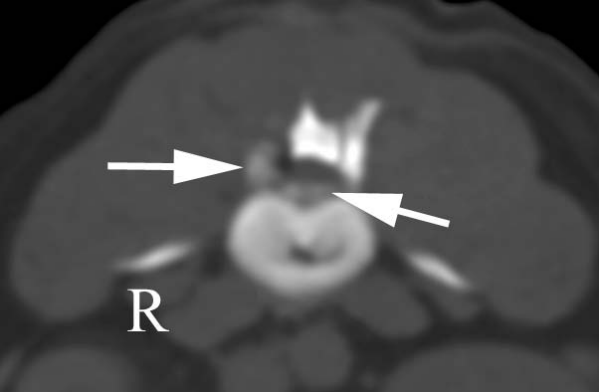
CT scan in bone window through L3-4 four days after right sided hemilaminectomy. The scan reveals calcified material (arrows) ventrally and laterally to the right of a compressed spinal cord. There is less calcification of the intervertebral disc compared to Figure 5. These findings suggest a recurrent disc extrusion that was confirmed at surgery.
